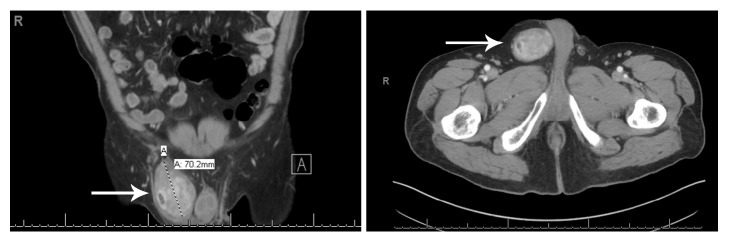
Computed tomography (CT) scans revealing a partially visualized, heterogenous enhancing right inguinal mass (arrows).
